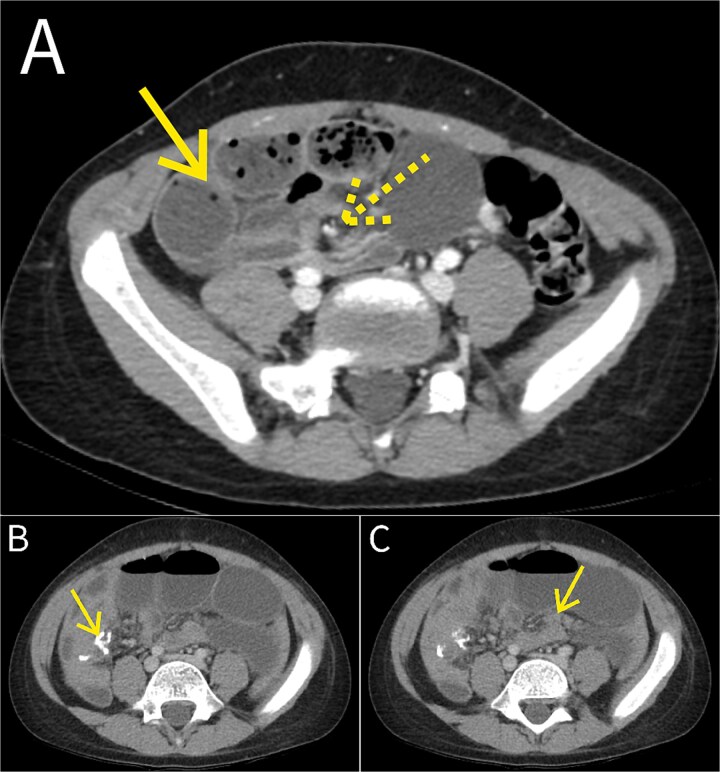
CT findings. (A) Contrast-enhanced CT of the abdomen and pelvis at the outside hospital demonstrating distended distal small-bowel loops with faecalization and mesenteric swirl, concerning for high-grade small bowel obstruction (SBO). The dashed arrow indicates the mesenteric swirl, and the solid arrow indicates a dilated distal small-bowel loop. The appendix is not dilated. (B) Postoperative contrast-enhanced CT obtained after recurrence of obstructive symptoms demonstrating the stapled small-bowel anastomosis (arrows). (C) Postoperative contrast-enhanced CT demonstrating a transition point in the right abdomen a few centimeters medial to the anastomosis (arrows), with marked proximal small-bowel dilatation and decompressed distal small bowel and colon, consistent with high-grade mechanical SBO. No abscess or anastomotic leak is identified.
